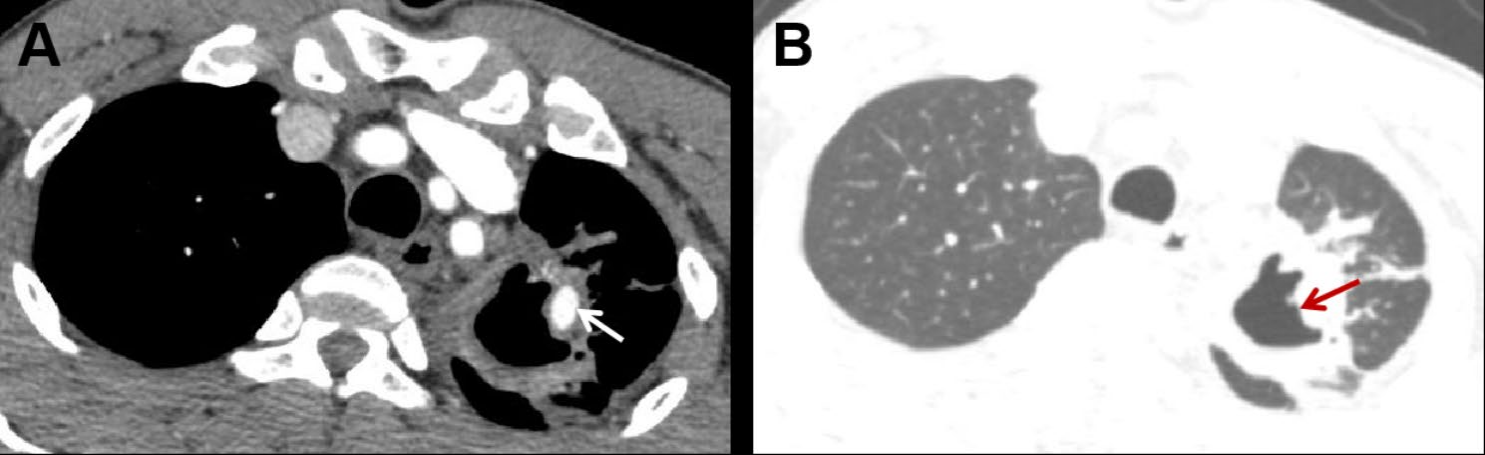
Representative images of tuberculous cavity combined with pulmonary artery pseudoaneurysm. A 54-year-old male was admitted to the hospital due to massive hemoptysis. He was diagnosed with sputum smear-positive pulmonary tuberculosis. (A) Axial contrast-enhanced CT scan shows a pseudoaneurysm (arrow) in the segmental pulmonary artery of the left upper lobe; (B) Axial contrast-enhanced CT scan shows a large cavitary lesion (red arrow) adjacent to the PAP
